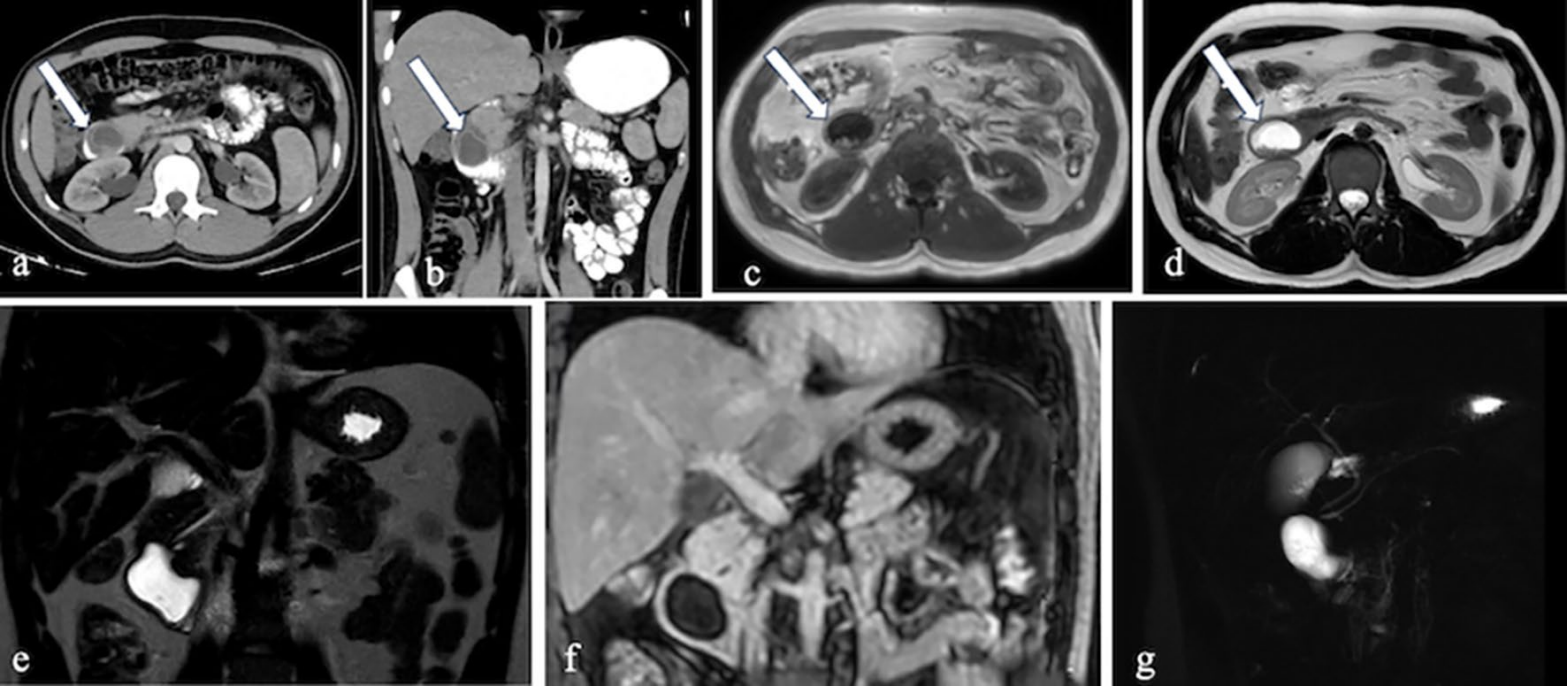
a. Axial and b. Coronal CT images with positive oral and intravenous (IV) contrast administration, c–g MRI images of a 25-year-old male patient with a duodenal duplication cyst, including axial T1-weighted (c), axial T2-weighted (d), coronal T2-weighted (e), coronal post-contrast fat-saturated T1-weighted (f), and MRCP (g) sequences. The cystic lesion exhibits no contrast enhancement. Multiple millimetric hypointense signals, consistent with millimetric stones, are observed on MRI. MRCP reveals no connection with the pancreaticobiliary system (arrows).
